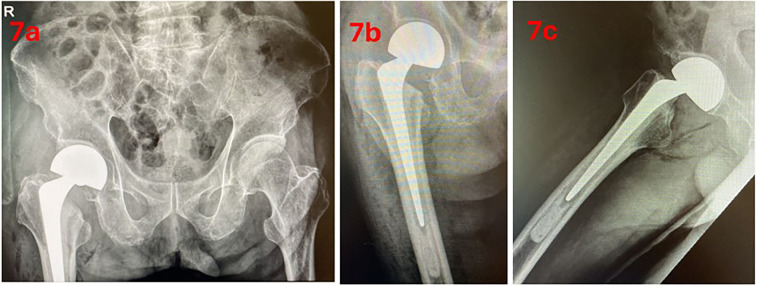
Post-operative radiographs; (a) anteroposterior pelvis view, (b) anterior hip view, (c) lateral hip view.
